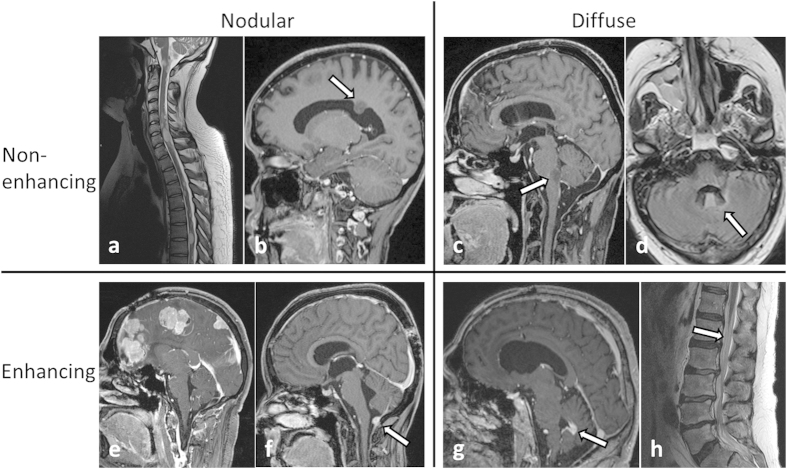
MRI examples of LM in the study population (a–c and e–h: T1w +Gd, d: T2-FLAIRw MRI). Two general morphological types of LM – nodular (a+b, e+f) or diffuse (c+d, g+h) – were observed. Both types occurred as enhancing (lower row) or non-enhancing (upper row) lesions. White arrows indicate sites of LM.
